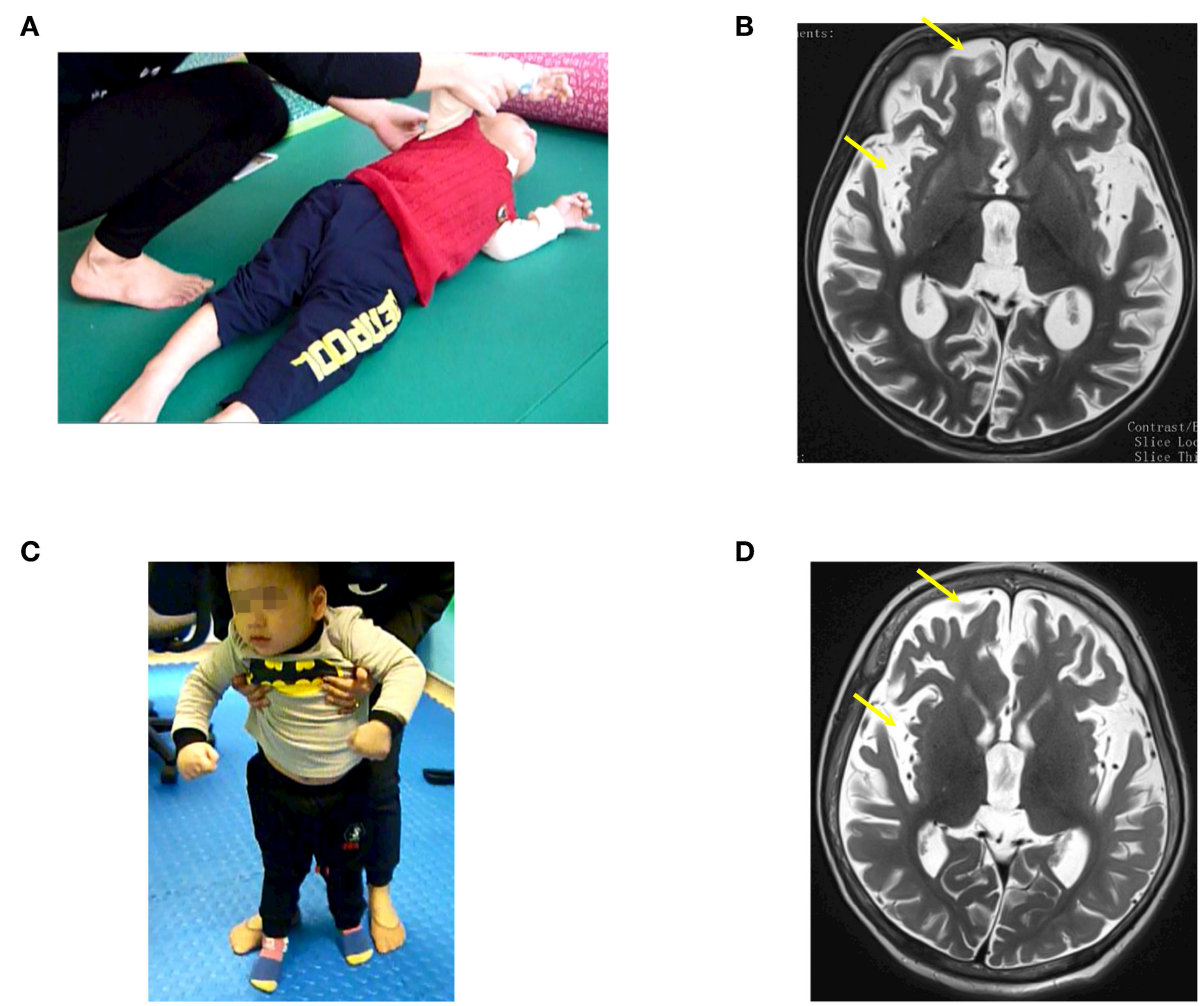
The patient (case 1) before and after autologous bone marrow-derived mononuclear cell (BMMNC) transplantation. (A) The patient before BMMNC transplantation is in a VS 3 months after the drowning. He had no awareness, no motor function, and exhibited muscle spasticity at level V. (B) Brain MRI demonstrated diffuse cerebral atrophy (arrows) at supratentorial and infratentorial regions, abnormal signal intensity at bilateral cortical parietal lobe, and bilateral putamen. (C) Six months after the third transplantation, the patient could express his need by vocalization, smile during communication, and stand. (D) No abnormal signal at bilateral cortical parietal lobe and bilateral putamen was observed at 12 months after the third transplantation.
